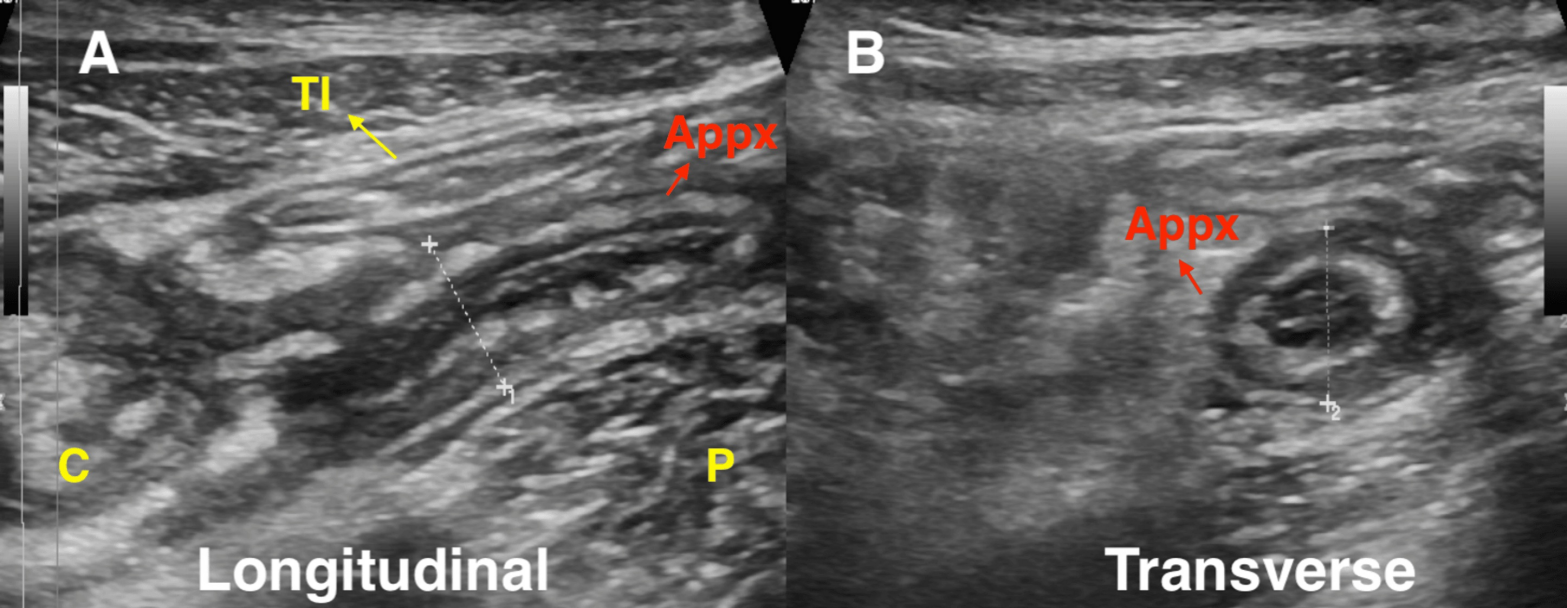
Visualization of the inflamed appendix in USG. (A) The longitudinal axis shows a dilated, tubular, aperistaltic, non-compressible structure in the right iliac fossa arising from the cecum. (B) The transverse axis shows a distended appendix giving a target-like appearance. Original USG image of a patient diagnosed as AA with impending perforation. USG: ultrasound; AA: acute appendicitis; Appx: appendix; C: cecum; P: psoas; TI: terminal ileum
